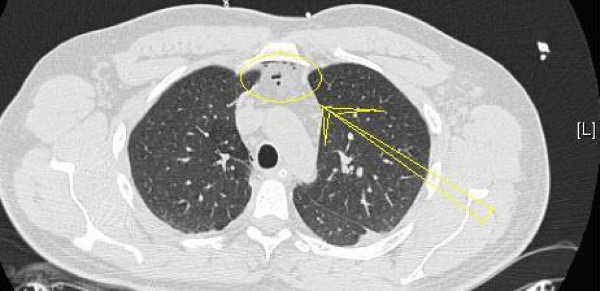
CT thorax of this patient demonstrating pneumomediastinum.
